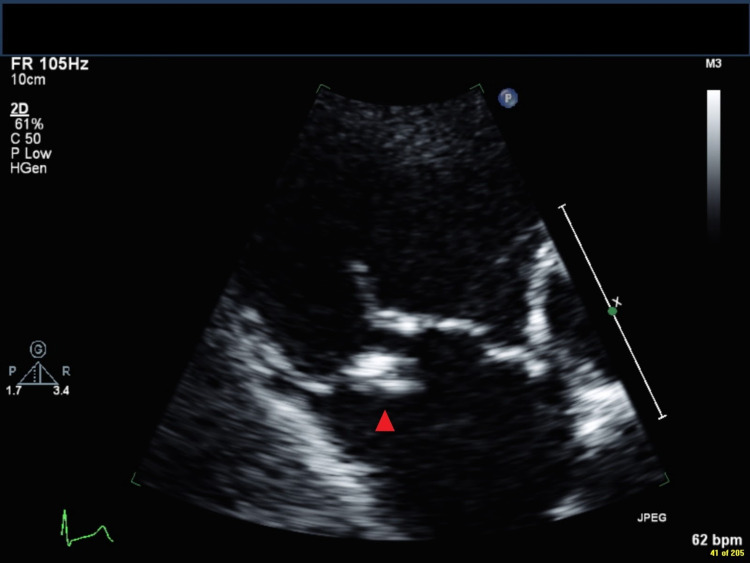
Transthoracic echocardiogram in parasternal right ventricular inflow view showing the vegetation (red arrowhead), which has increased in size to 11 × 6 mm, adherent to the atrial aspect of the tricuspid septal leaflet.
